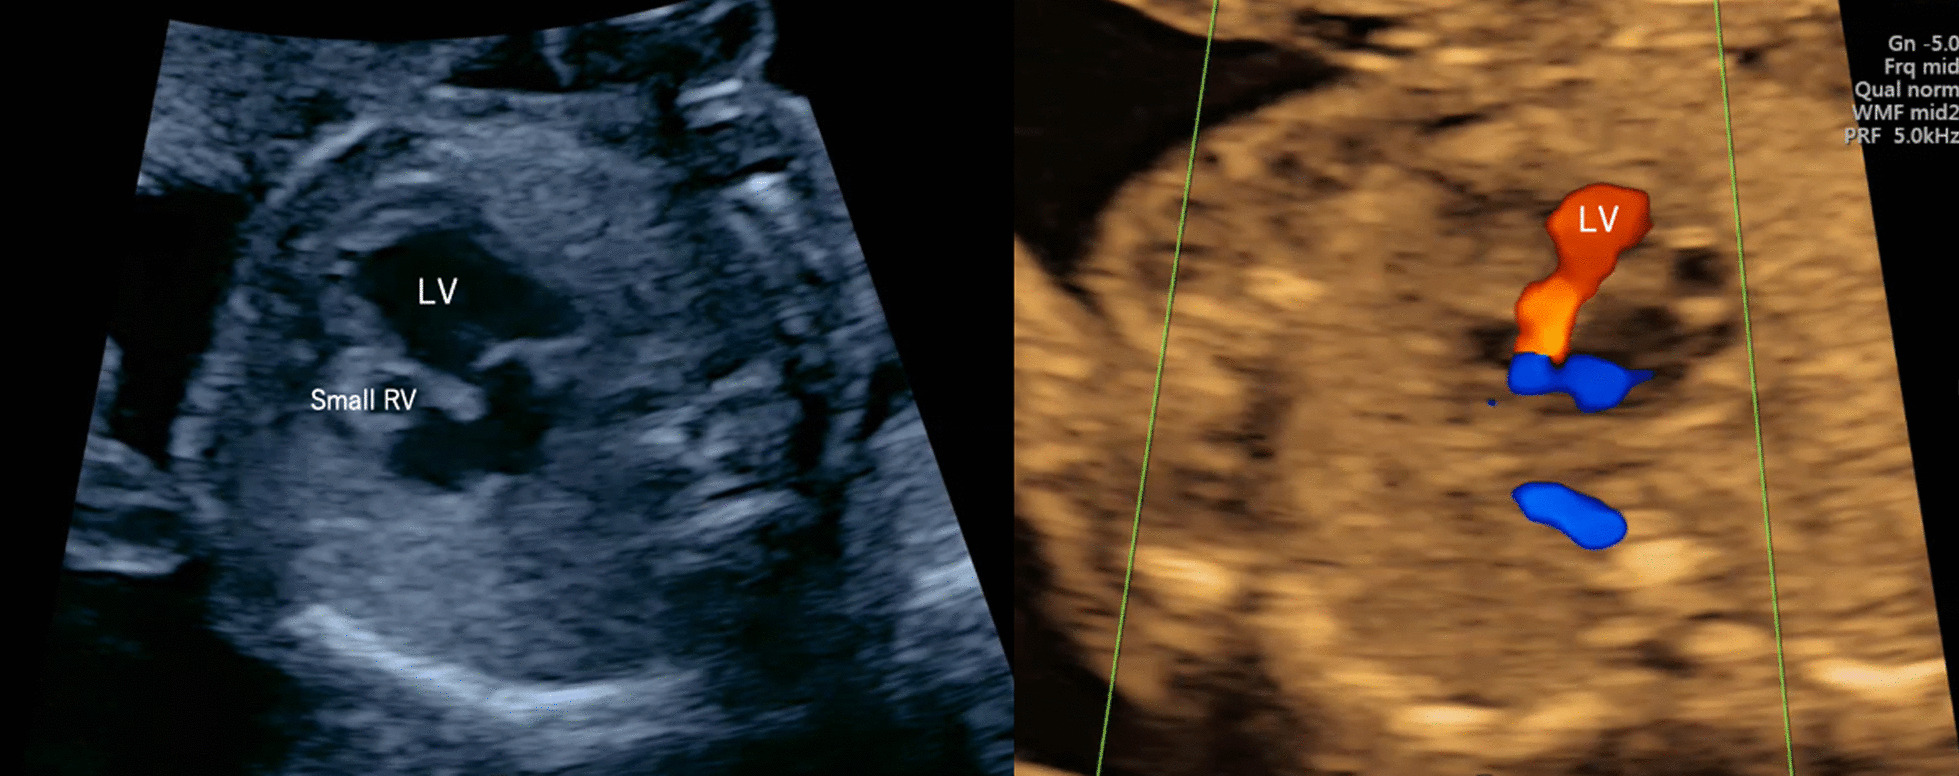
Fetal ultrasound images; 21 weeks and 1 day of gestation in the first pregnancy. Left image (B-mode): there was a left ventricular-type single ventricle. Right image (Color doppler): the pulmonary artery was closed and we observed the mitral regurgitation. LV, left ventricle; RV, right ventricle
